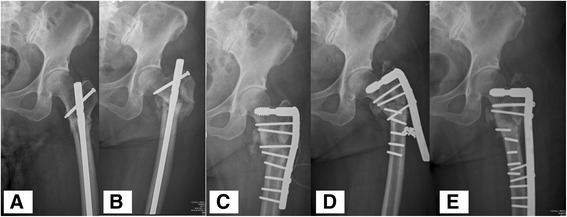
Another patient, who had a hypertension, diabetes mellitus, and breast cancer history, received bisphosphonates for 5 years. Left thigh pain developed after a minor trauma, and left atypical subtrochanteric fracture was noted. a Internal fixation with intramedullary nail was performed. b The reduction was failure and implants back out at post-operative one year due to nonunion. c Revision surgery was then performed with a Dynamic Condylar Screw with allobone grafting. d Left thigh pain 4 months later with difficulty in weight-bearing. Radiography showed implant failure again with multiple broken screws. e Another revision surgery was performed with a longer Dynamic Condylar Screw with allobone grafting. The patient was under follow-up in the outpatient department
